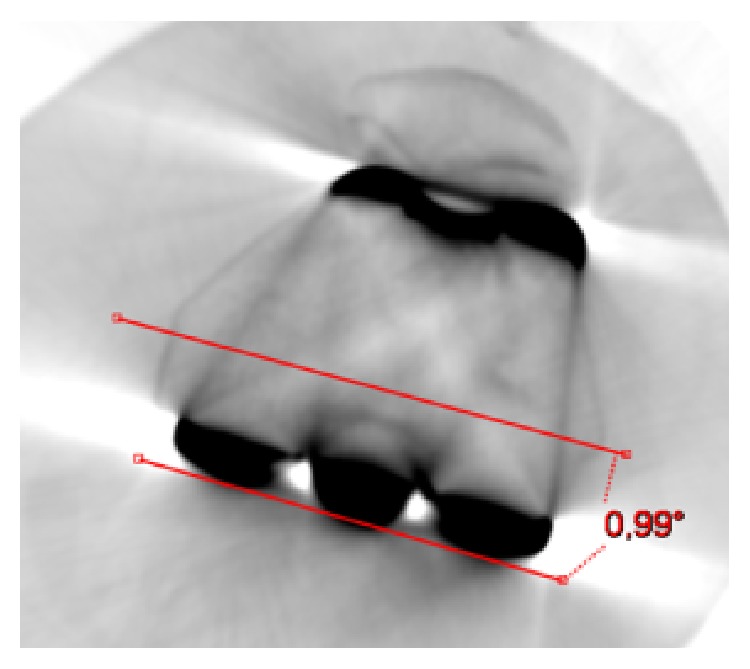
Left knee. Femoral component (Persona, The Personalized Knee System, Zimmer, Warsaw, USA) computed tomography (CT) axial view. The angle (PCA) between the surgical transepicondylar axis (sTEA) and the line of the posterior condyles is 0.99°.
